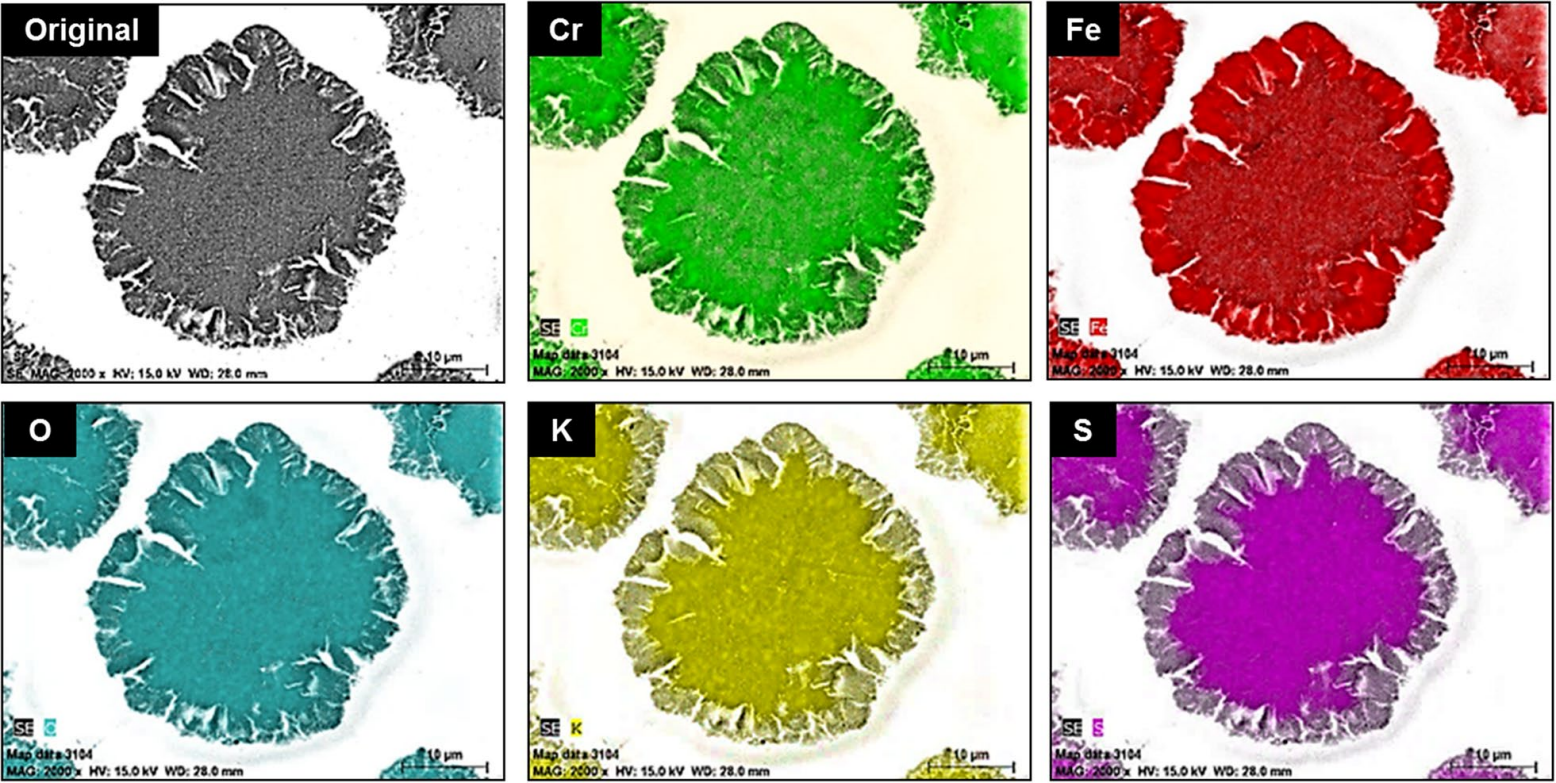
Energy dispersive X-ray mapping of a partially decomposed KFe_3_[(SO_4_)_2 − X_(CrO_4_)_X_](OH)_6_ particle in NaOH medium; pH 12.14, T = 30 °C (303 K), d_0_ = 38–44 µm, RPM = 750 min^−1^. The results confirm the unreacted core model and the adsorption of CrO_4_
